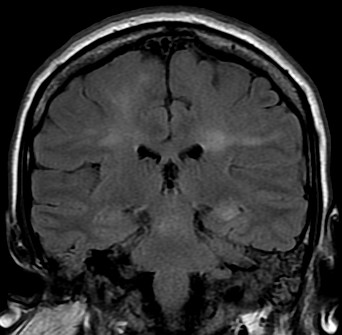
Magnetic resonance imaging scans of the brain acquired the day after readmission. The coronal fluid attenuation inversion recovery image shows several ill-defined foci of high T2 signal in the corona radiata of both hemispheres.
